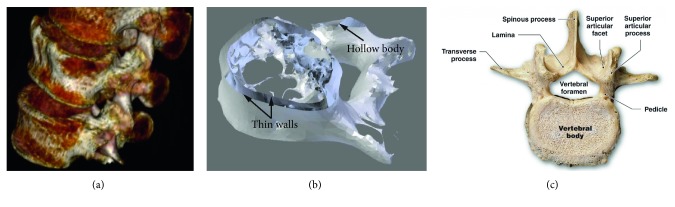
(a) CT scan of the lower spine showing the L3 (top), L4 (middle), and L5 (bottom) lumbar vertebrae [32]. (b) View of the STL file of an L5 lumbar vertebra from [32] exhibiting infinitesimally thin walls and a hollow body. (c) Anatomy of the lumbar vertebrae [33].
